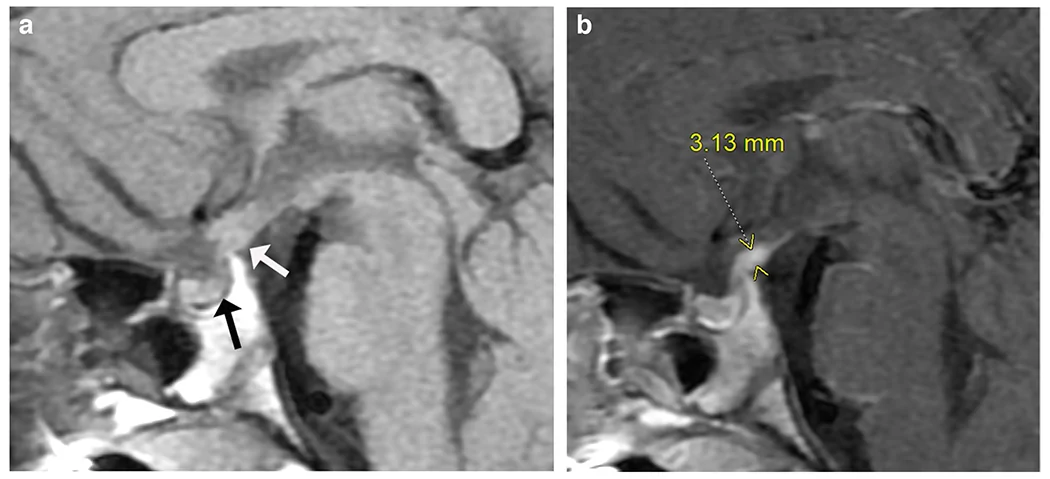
Ten-year-old male with central diabetes insipidus. a Sagittal T1-weighted brain MRI shows absence of the posterior pituitary bright spot (*black arrow* in a); no ectopic bright spot was present, but the isointense pituitary infundibulum appears thickened (*white arrow* in a). b Post-contrast image shows 3.1-mm thickening of the upper pituitary infundibulum (*calipers* in b). Differential considerations include infundibular-neurohypophysitis, IgG4 hypophysitis, Langerhans cell histiocytosis, and early manifestation of germ cell tumor. Tumor markers and cerebrospinal fluid assessment were normal. Etiology was presumed inflammatory. The patient was managed conservatively with repeat brain MRI exams over the following 2 years to exclude development of neoplasm (not shown)
